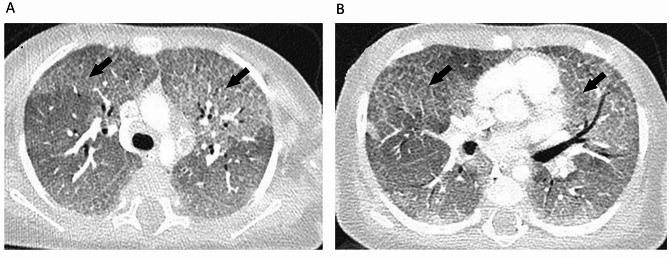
A CT scan of the chest revealed bilateral, diffuse ground-glass opacities with crazy paving pattern (arrows)
